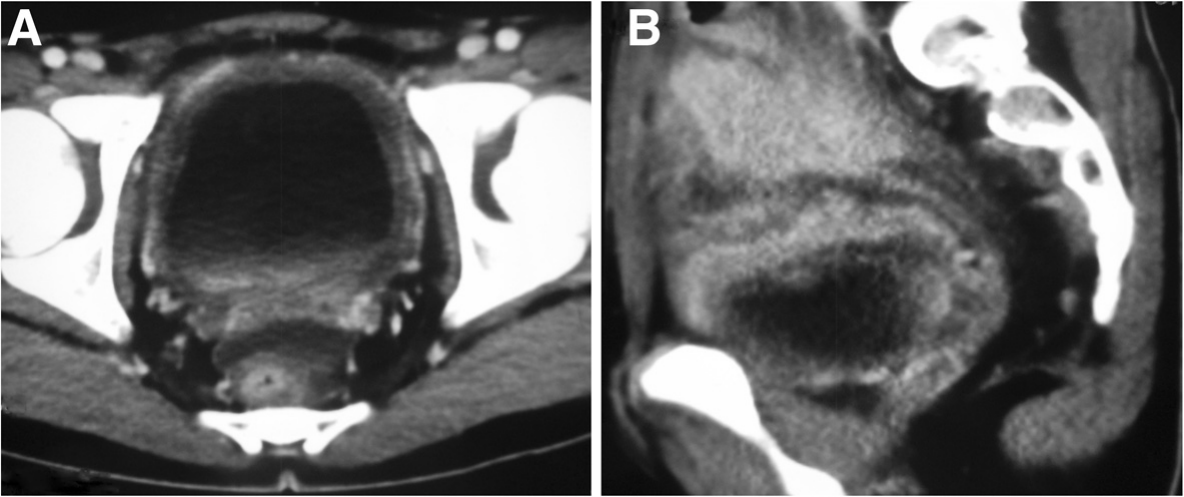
Enhanced computed tomography scan of the pelvis. (A) Enhanced computed tomography (CT) scan of the pelvis showing uneven thickening of the bladder wall and heterogeneous enhancement performance. (B) Enhanced CT revealing infiltration by a large, irregular, neoformative mass involving the adjacent peritoneum and ileocecal junction.
